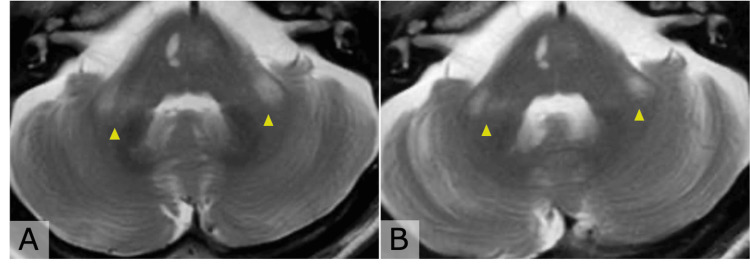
T2-weighted MRI showing reversible hyperintense lesions in the middle cerebellar peduncles before and after corticosteroid therapy. (A) Pre-treatment image demonstrating bilateral hyperintense lesions in the middle cerebellar peduncles (yellow arrowheads). (B) Post-treatment image showing a reduction in the hyperintense lesions (yellow arrowheads).
